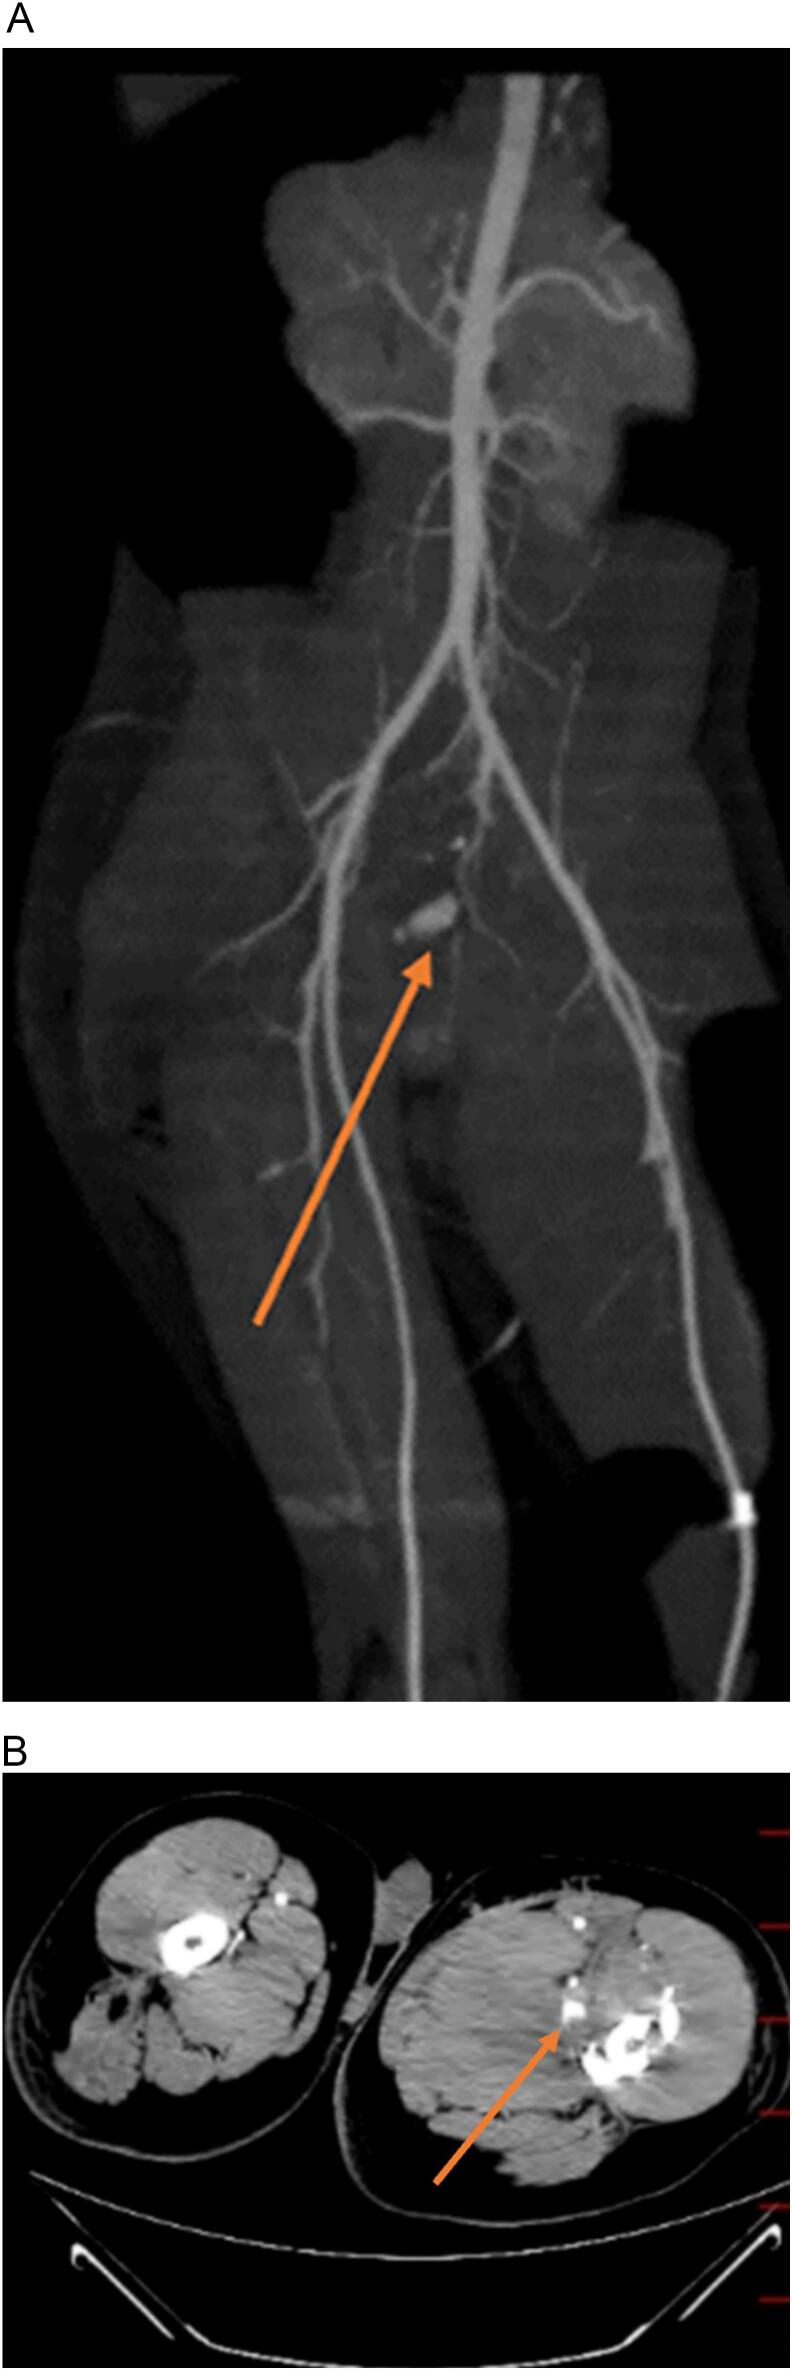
CT angiography revealed a pseudoaneurysm of the deep femoral artery. A: Coronal view; B: Axial view.
